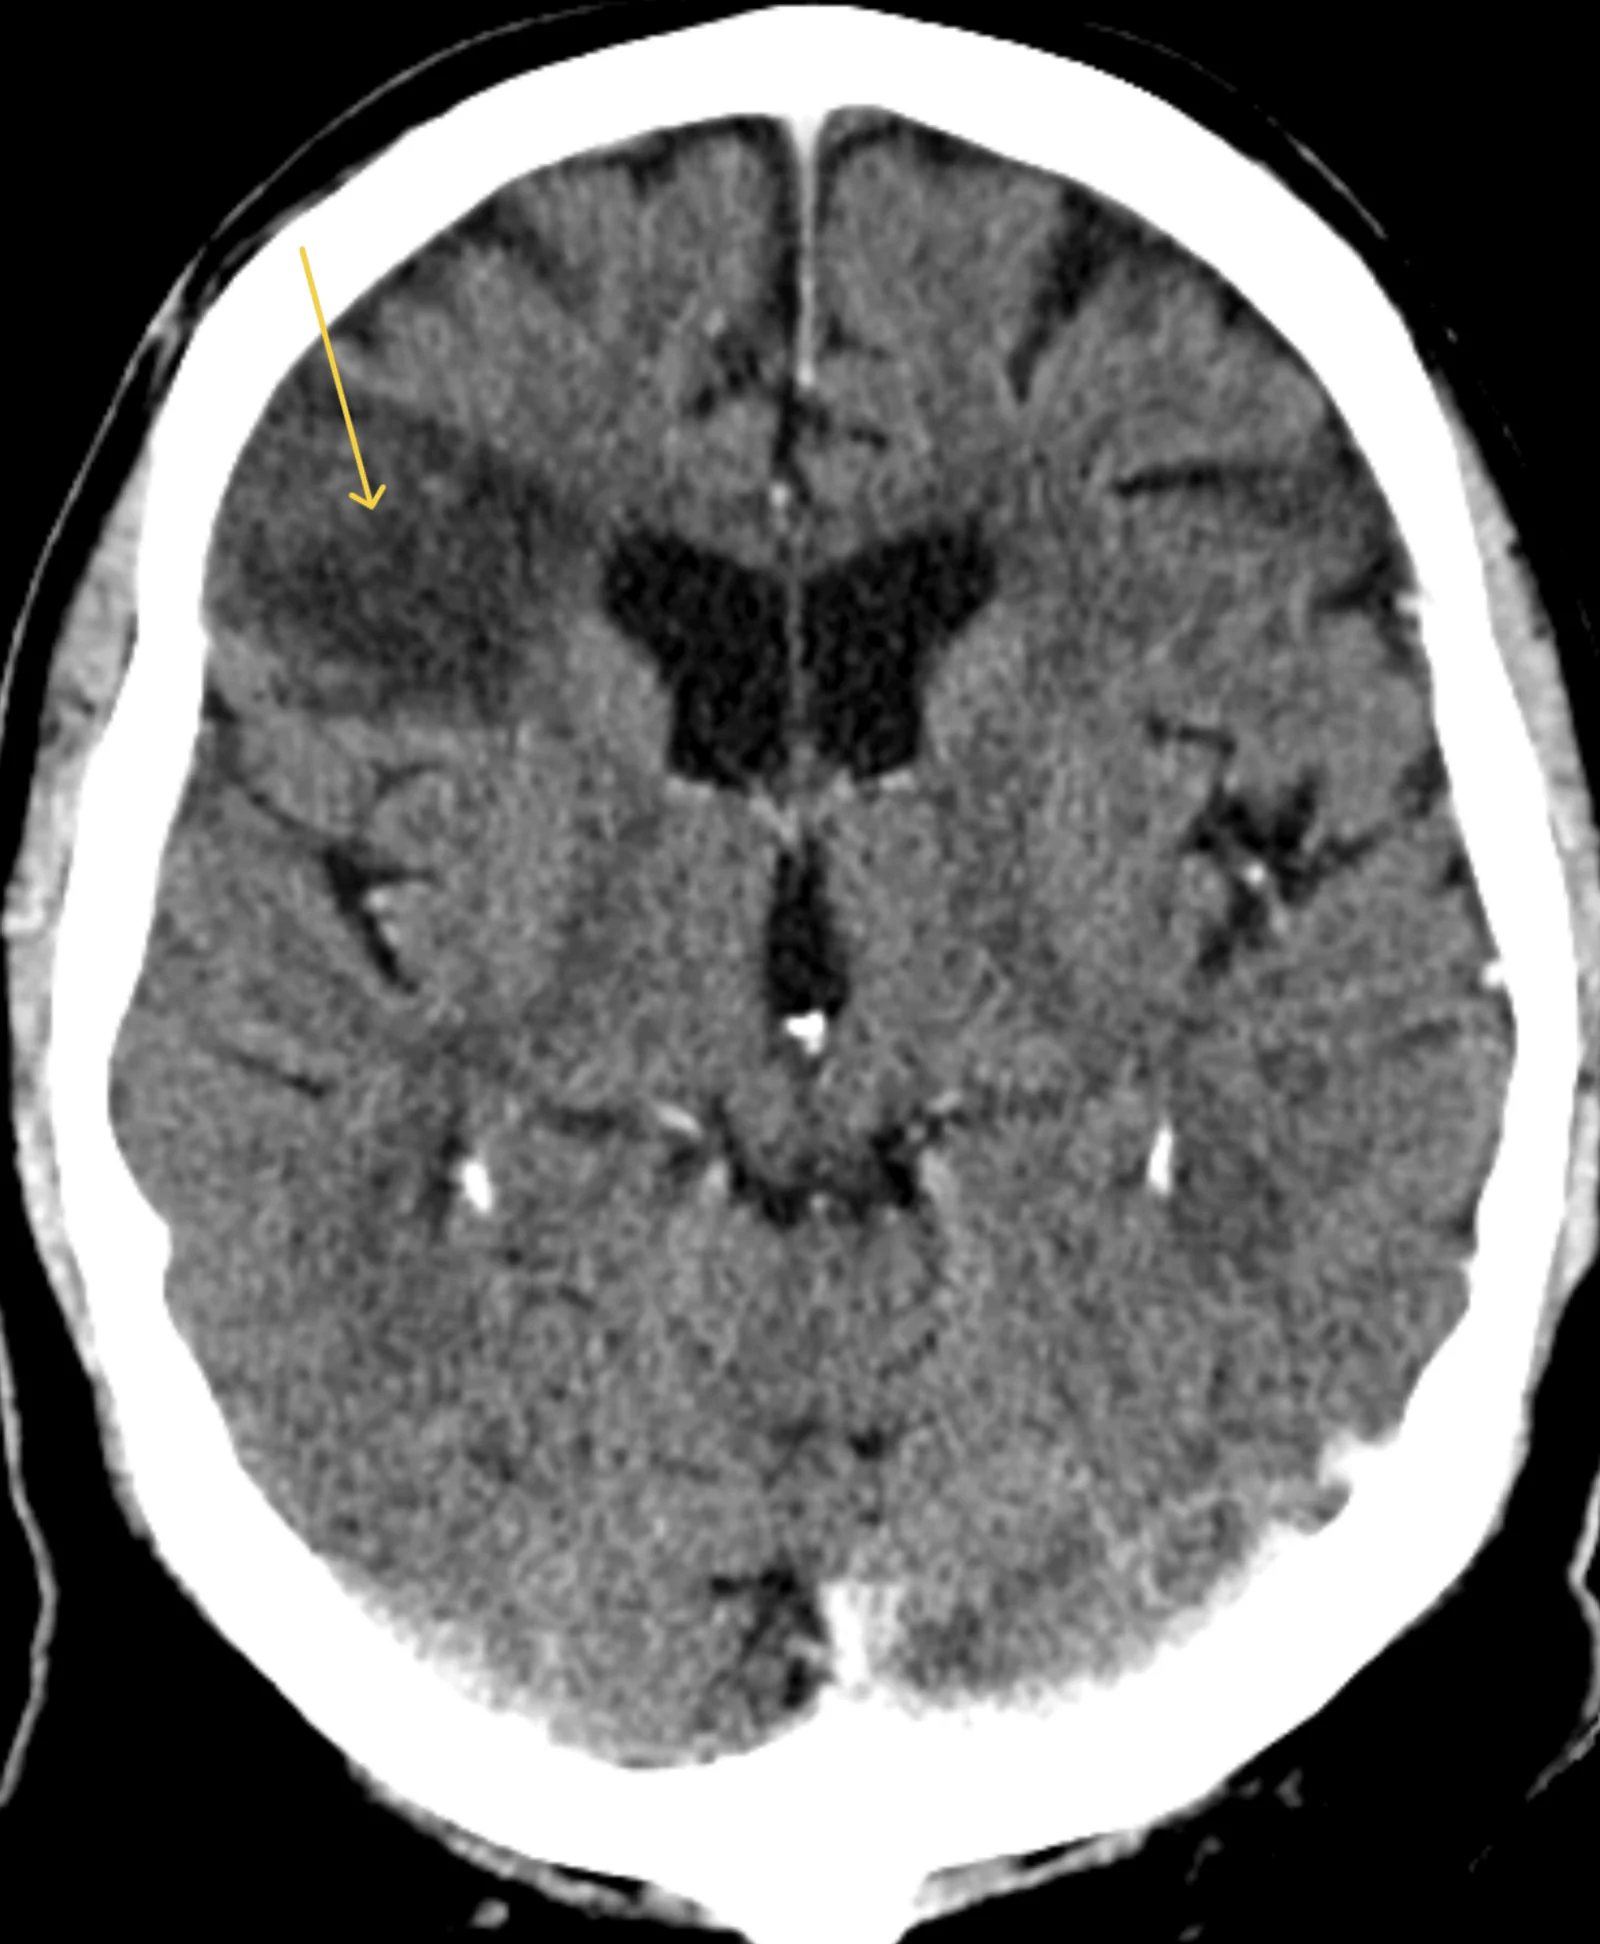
Axial non-contrast CT of the head on Day 1 demonstrating an approximately 3 cm focus of hypoattenuation in the right frontal lobe (yellow arrow), initially interpreted as an infarction of indeterminate age versus a neoplastic lesion CT: computed tomography
